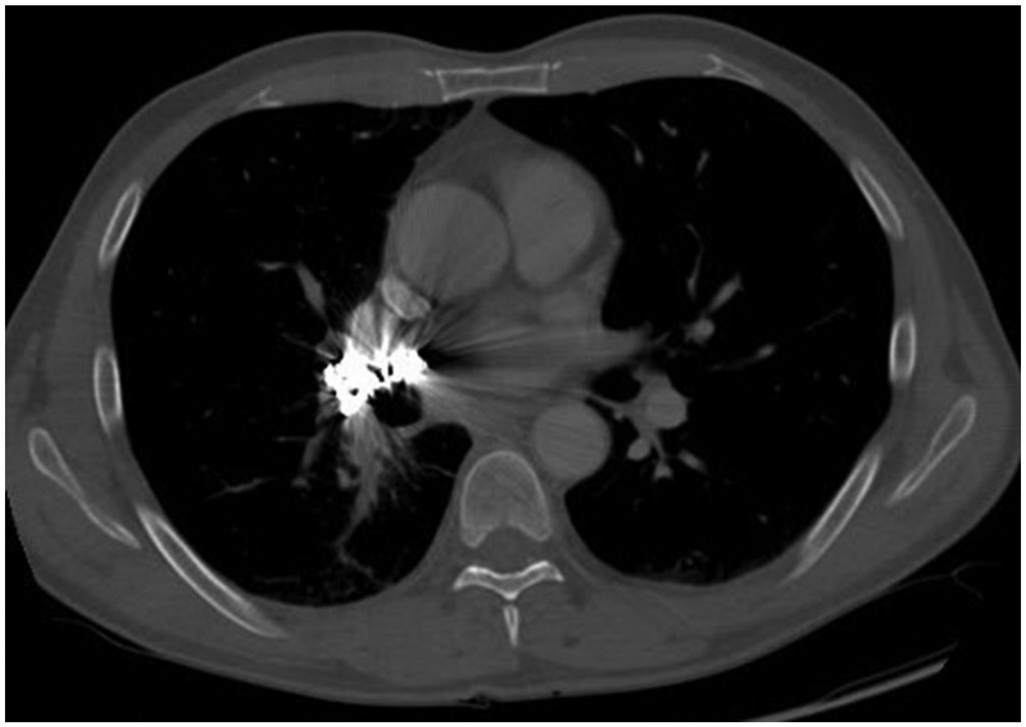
Thoracic CT scan image after the pulmonary artery embolisation procedure. Contrast-enhanced CT image with soft-tissue window showing multiple coils embolised to the right main pulmonary artery, as well as a partially decreased tumour and reduced occlusion of the right lower lobe bronchus.
